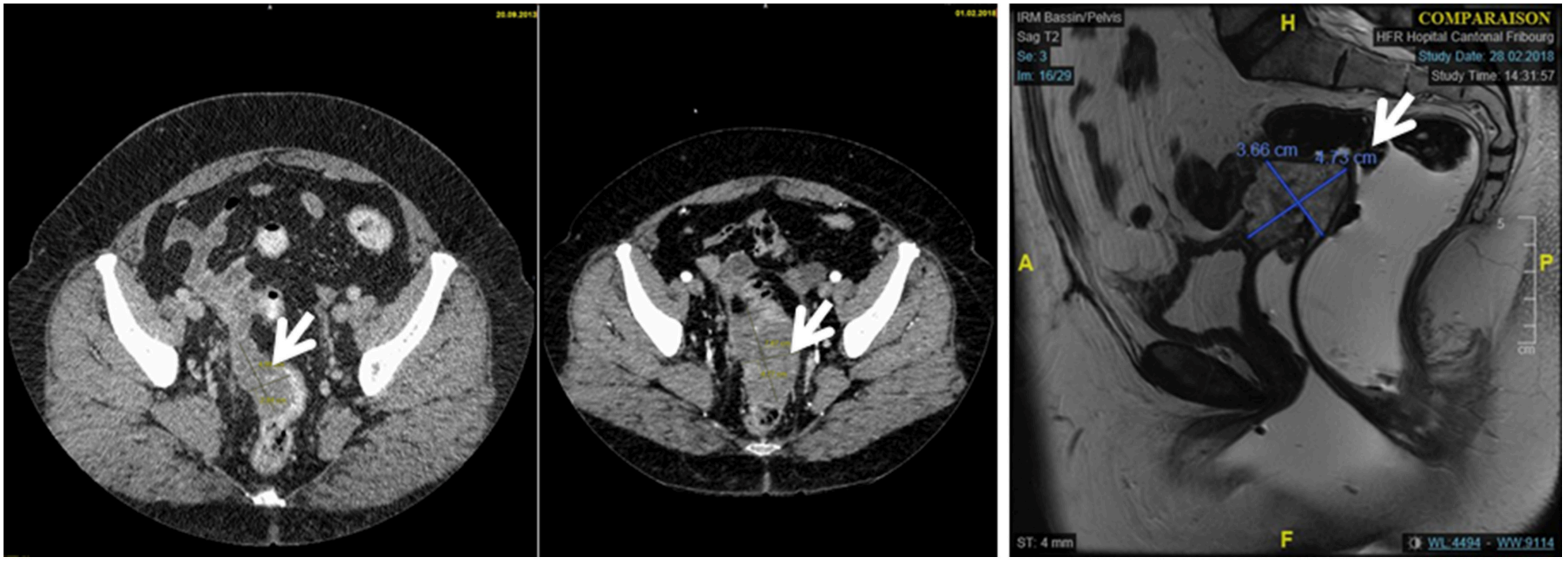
Comparison of the 2013 and 2018 CT scanner showing an enlargement of the vaginal mass from 48 x 24 mm to 76 x 47 mm.
